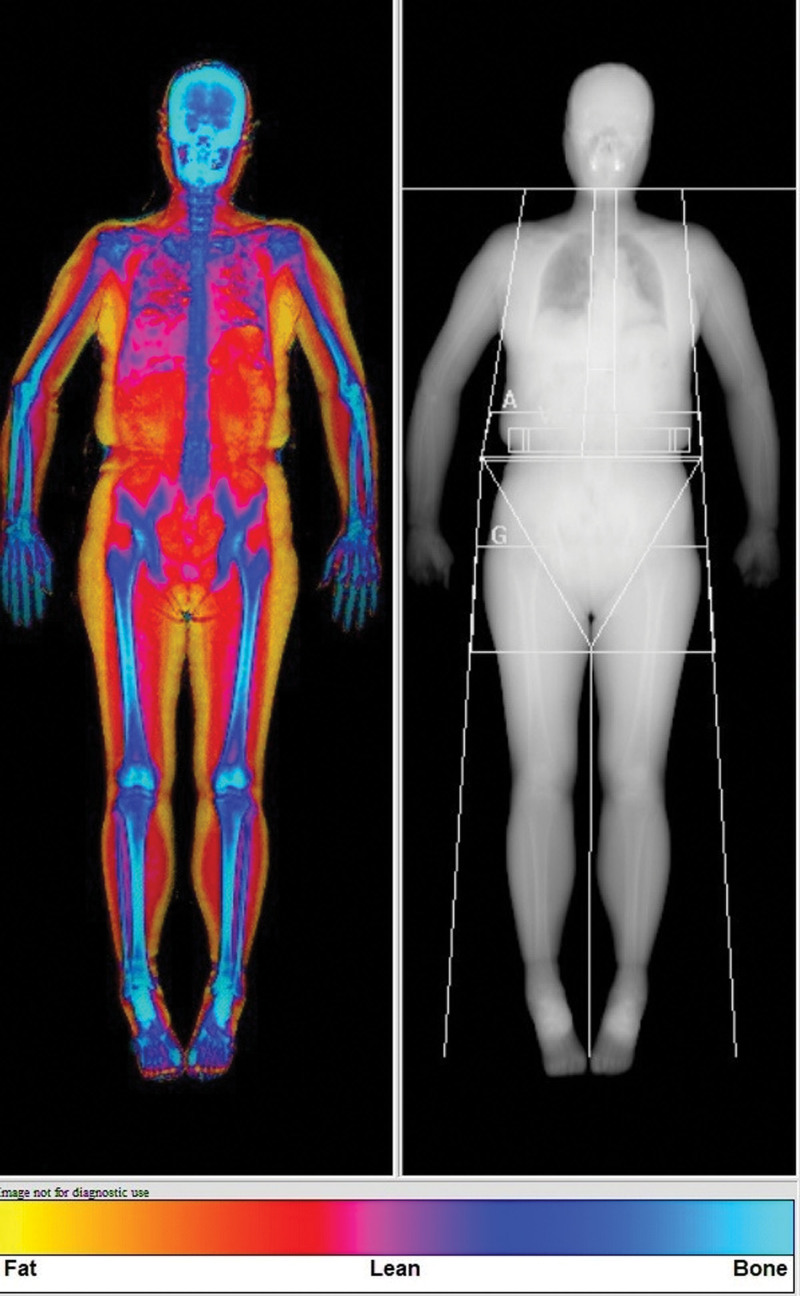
Example of dual-energy X-ray absorptiometry for muscle (lean) mass and bone density.
